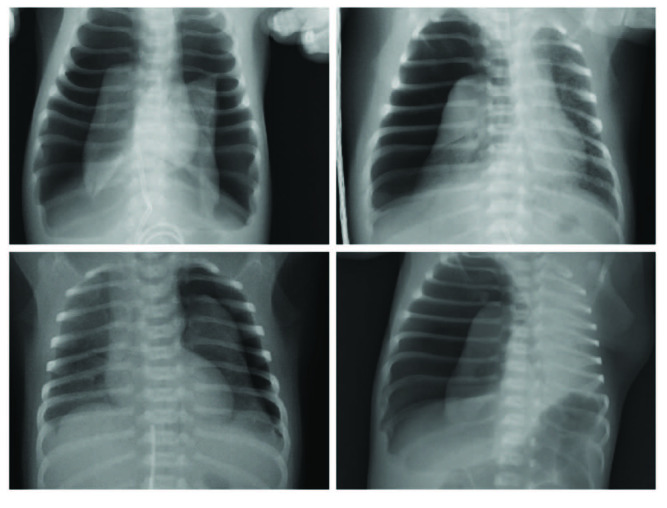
Radiographs of patients with type I pneumothorax.
